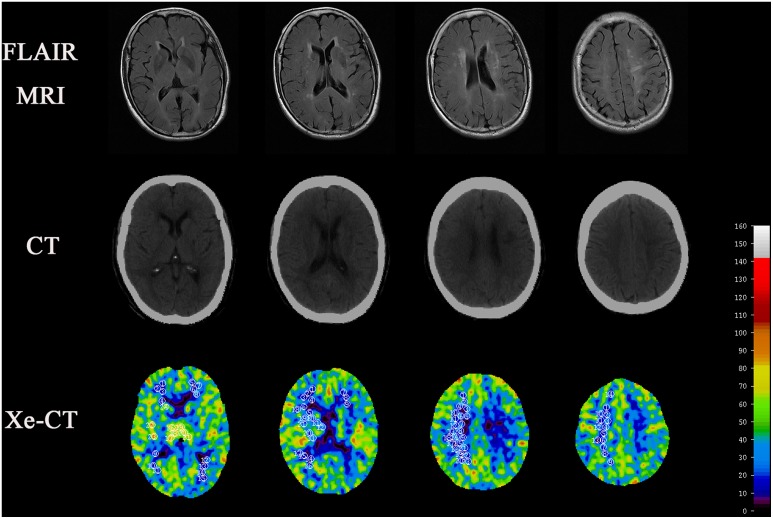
Flair imaging, normal CT and Xe-CT at different levels of subjects with WML.
